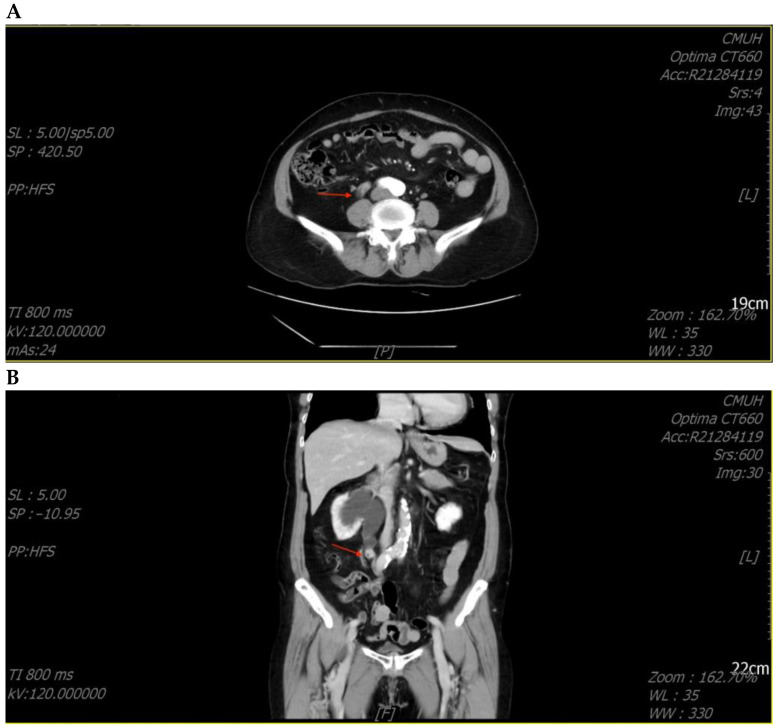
Computed tomography of the abdomen revealed a tumor (arrowhead) in the right upper ureter with proximal dilatation. (A) Coronary view; (B) Sagittal view.
